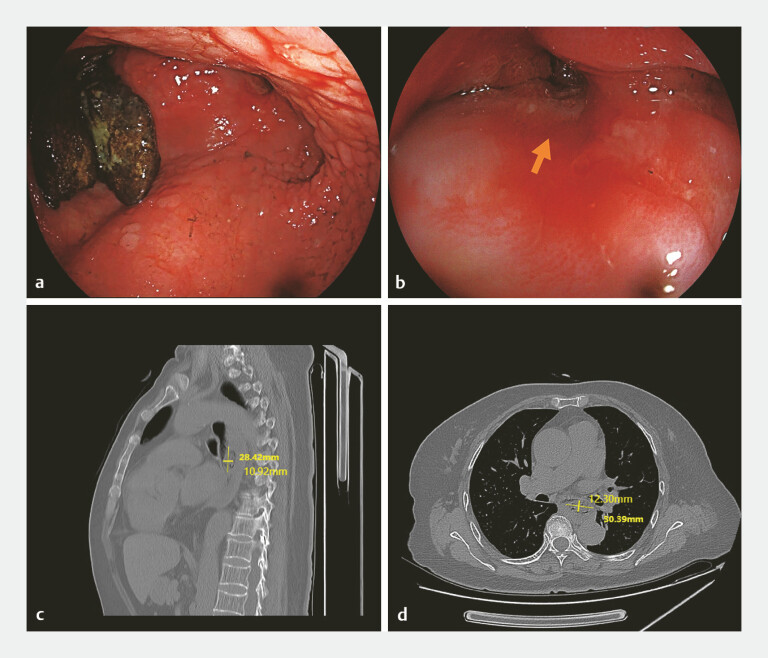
Pre-procedural localization of esophageal diospyrobezoars by endoscopy and CT.
